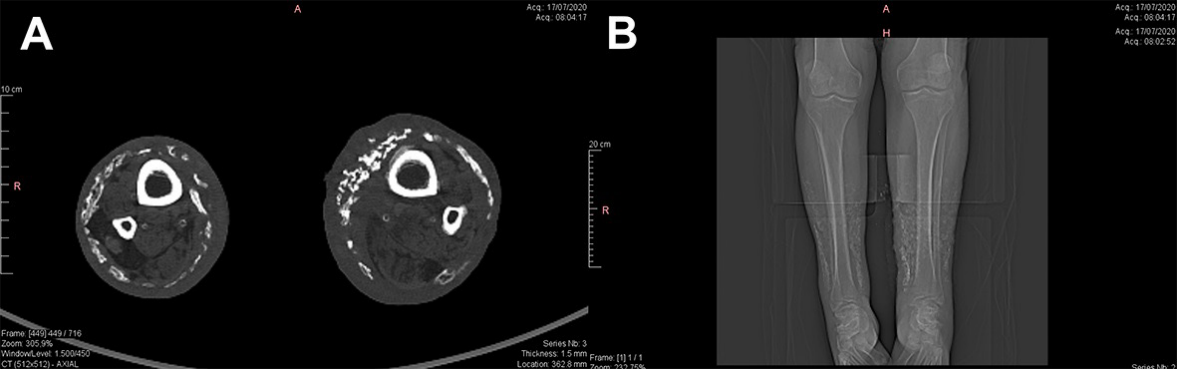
A) Computed tomography of the lower limbs (transverse and longitudinal slices) showing diffuse calcium deposits distributed concentrically around the mid and distal thirds of both lower limbs, with no deposits present in other regions of the body.
